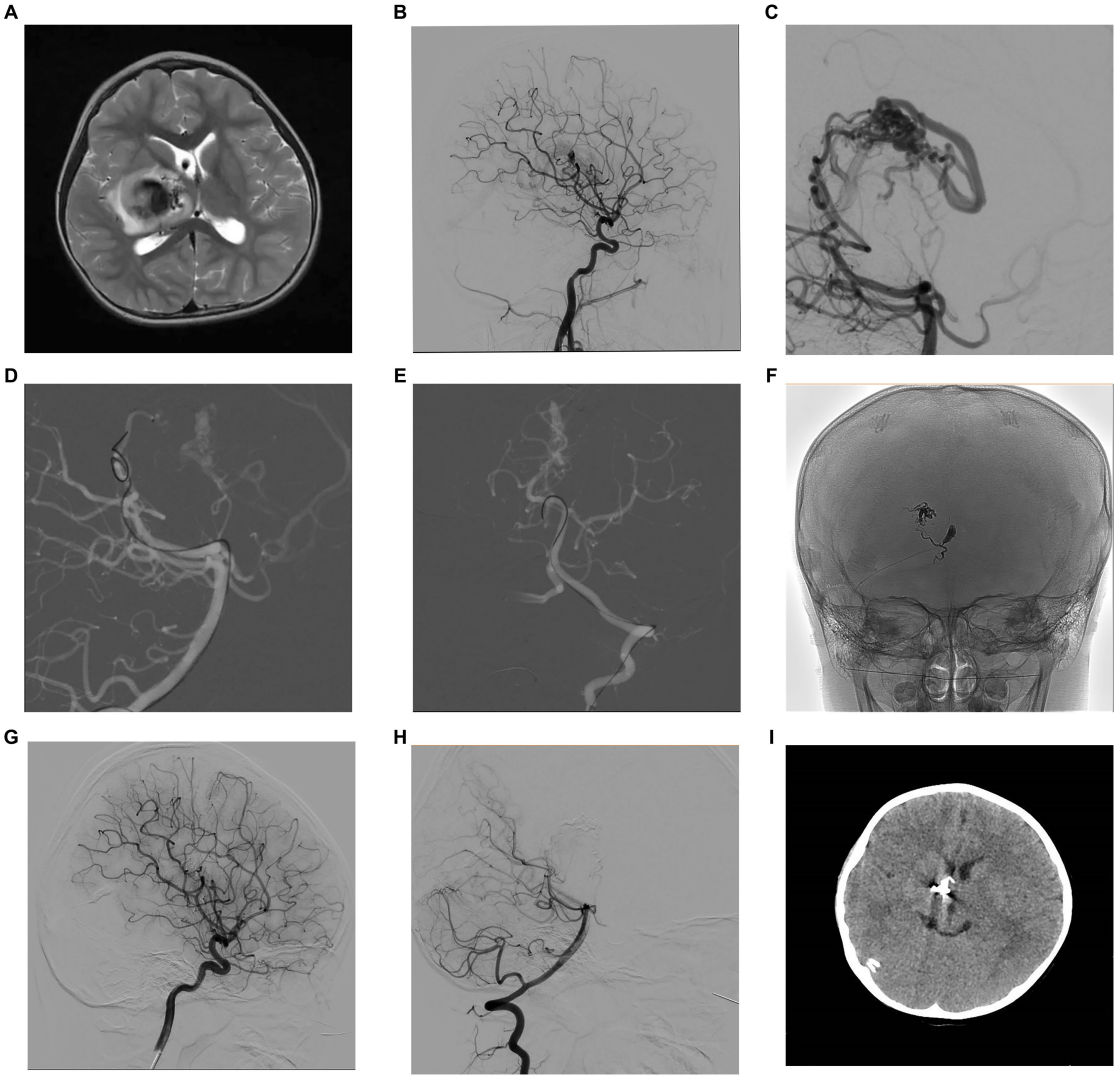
A 4-year-old female patient presented with left limb weakness. Axial T2-weighted MRI (A) showed an AVM located in the right basal ganglia and thalamic area. Selective DSA of the right ICA in lateral (B) view and vertebral artery in lateral (C) projection unfolded an AVM supplied by the branches of posterior communicating artery and posterior cerebral artery, with a nidus measuring 1.3 cm and single deep venous drainage through the internal cerebral vein. Intraoperative road-map image of the vertebral artery in lateral (D) and AP (E) views exhibit the process of navigating to the posterior cerebral artery and the posterior communicating artery, respectively. Intraoperative nonsubtracted single shot imaging from the AP (F) view revealing the cast of Onyx (EV3, Irvine, California). After the intervention, control DSA within the right ICA in lateral (G) view and vertebral artery in lateral (H) view highlighted anatomical exclusion of nidus. Postoperative head CT (I) confirmed no intracranial hemorrhage and edema. AP, anteroposterior; AVM, arteriovenous malformation; CT, computed tomography; DSA, digital subtraction angiogram; ICA, internal carotid artery; MRI, magnetic resonance imaging.
